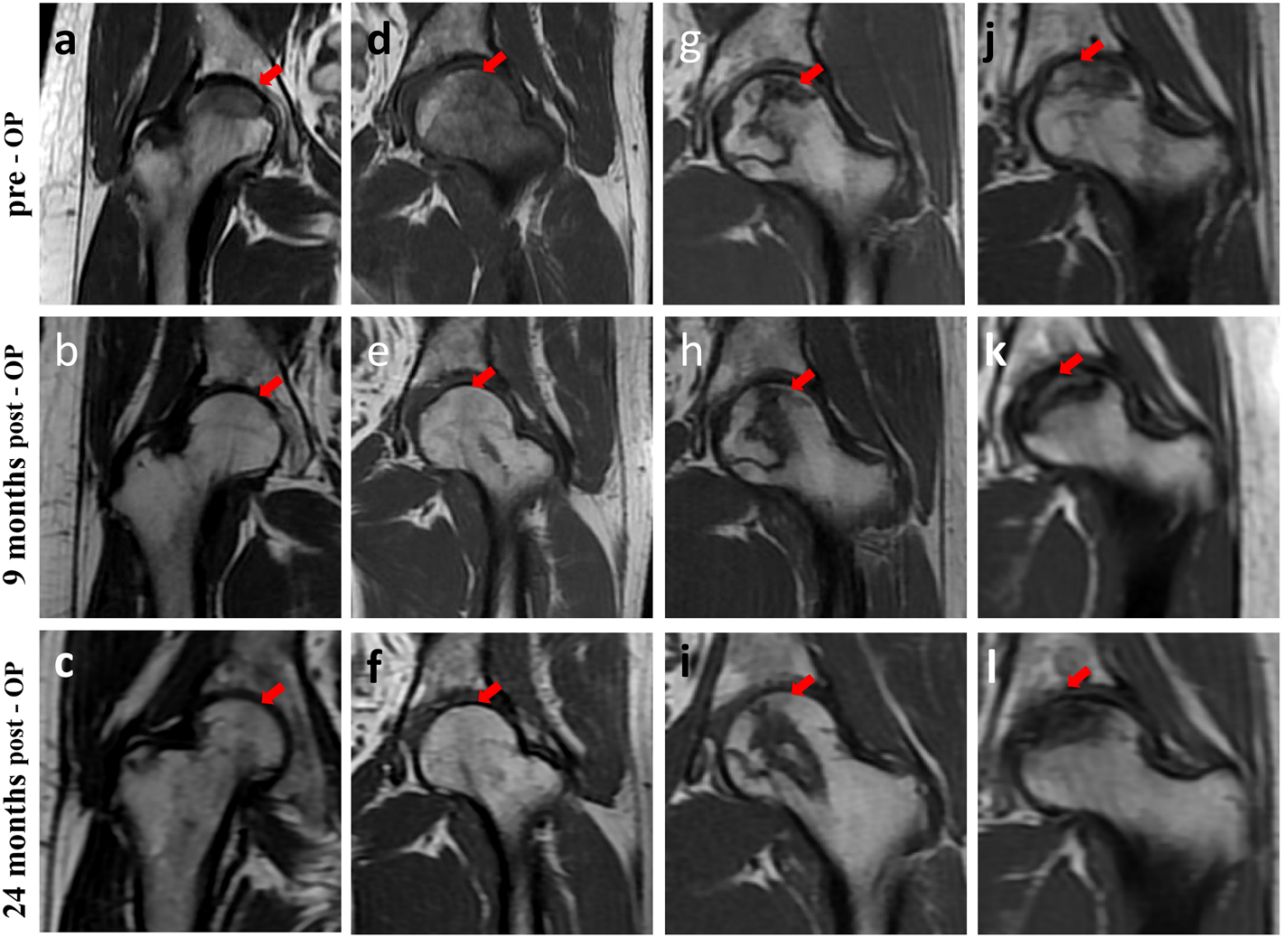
a-l T1-weighted MRI images of the necrotic area before and after the operation. Arrow Location of the necrotic area. a-f In some patients, the necrotic area ratio was significantly lower at 9 months after surgery than before surgery. g-l In other patients, the necrotic area ratio was not significantly lower at 9 months after surgery than before surgery. In all patients, the necrotic area did not change considerably from 9 months to 24 months after the operation
